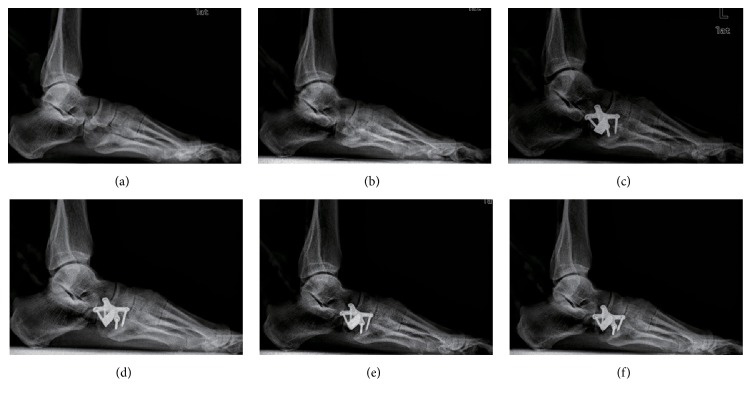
Lateral radiographs of six conditions: (a) intact foot, (b) flatfoot, and (c–f) lengthening of lateral column with cuboid osteotomy (2 mm, 3 mm, 4 mm, and 5 mm).
